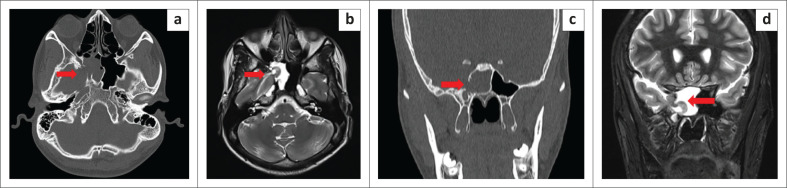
(a and c) Axial and coronal CT sections demonstrate a defect in the right lateral wall of the sphenoid sinus (red arrows). (b and d) Axial and coronal T2-weighted sequences reveal the herniated portion of right medial temporal lobe (red arrows).
